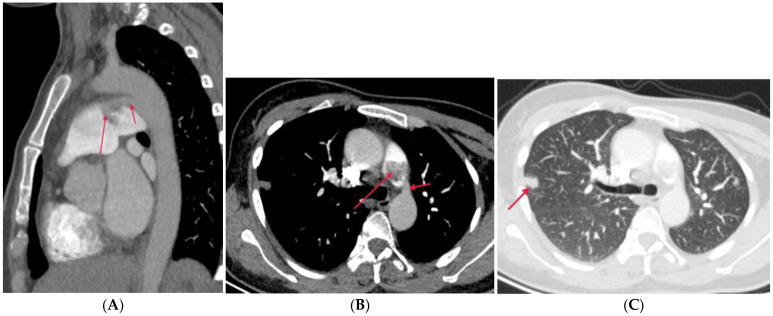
Septic pulmonary embolism in a 29-year-old woman with PDA and infective endocarditis. (A,B): Sagittal and axial CTPA images demonstrate a filling defect within the main pulmonary artery (long arrow) and at the end of the PDA (short arrow). (C): Axial CTPA on lung windowing shows sub-pleural opacity, consistent with peripheral infarction (arrow), which may be caused by the septic emboli. CTPA—computed tomography pulmonary angiography; PDA—patent ductus arteriosus.
